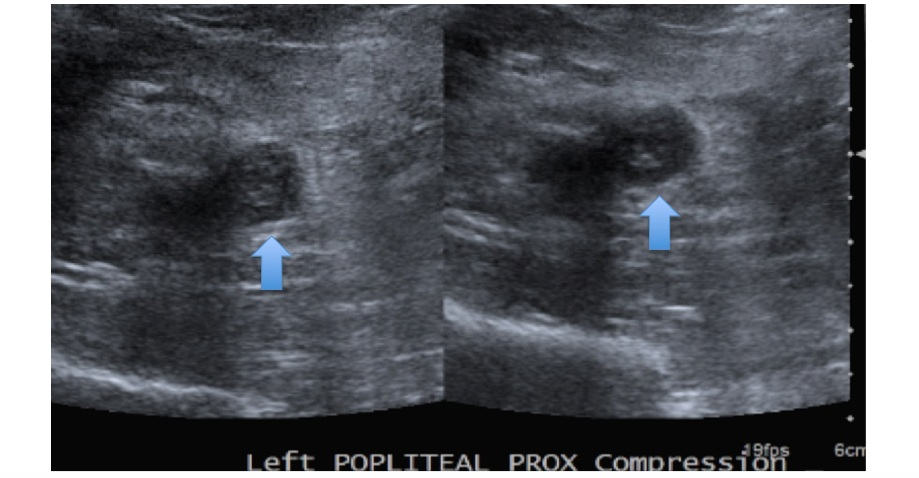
Ultrasound of DVT shows a noncompressible left popliteal vein
